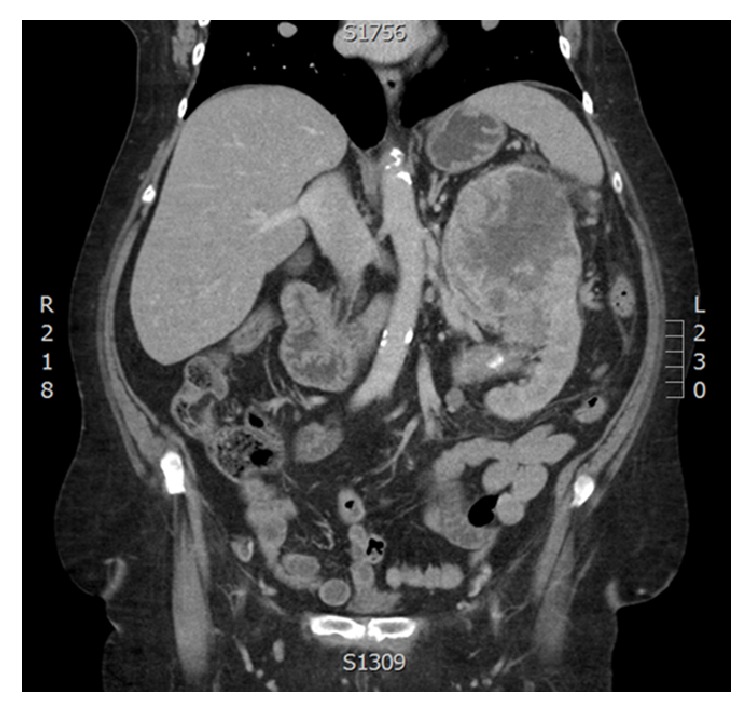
CT abdomen coronal view showing a large heterogeneous left renal mass with suspected splenic hilar invasion.
